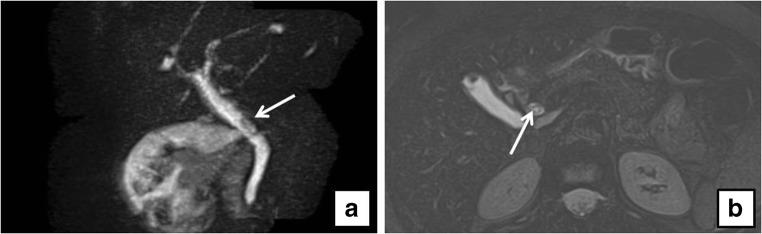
a Sagittal thick-slab magnetic resonance cholangio-pancreatography (MRCP) shows a filling defect in a dilated common bile duct (CBD) (*arrow*). b Axial fat-saturated T2-weighted magnetic resonance imaging (MRI) of the same patient shows a dishomogeneous filling defect in a dilated CBD (*arrow*)
